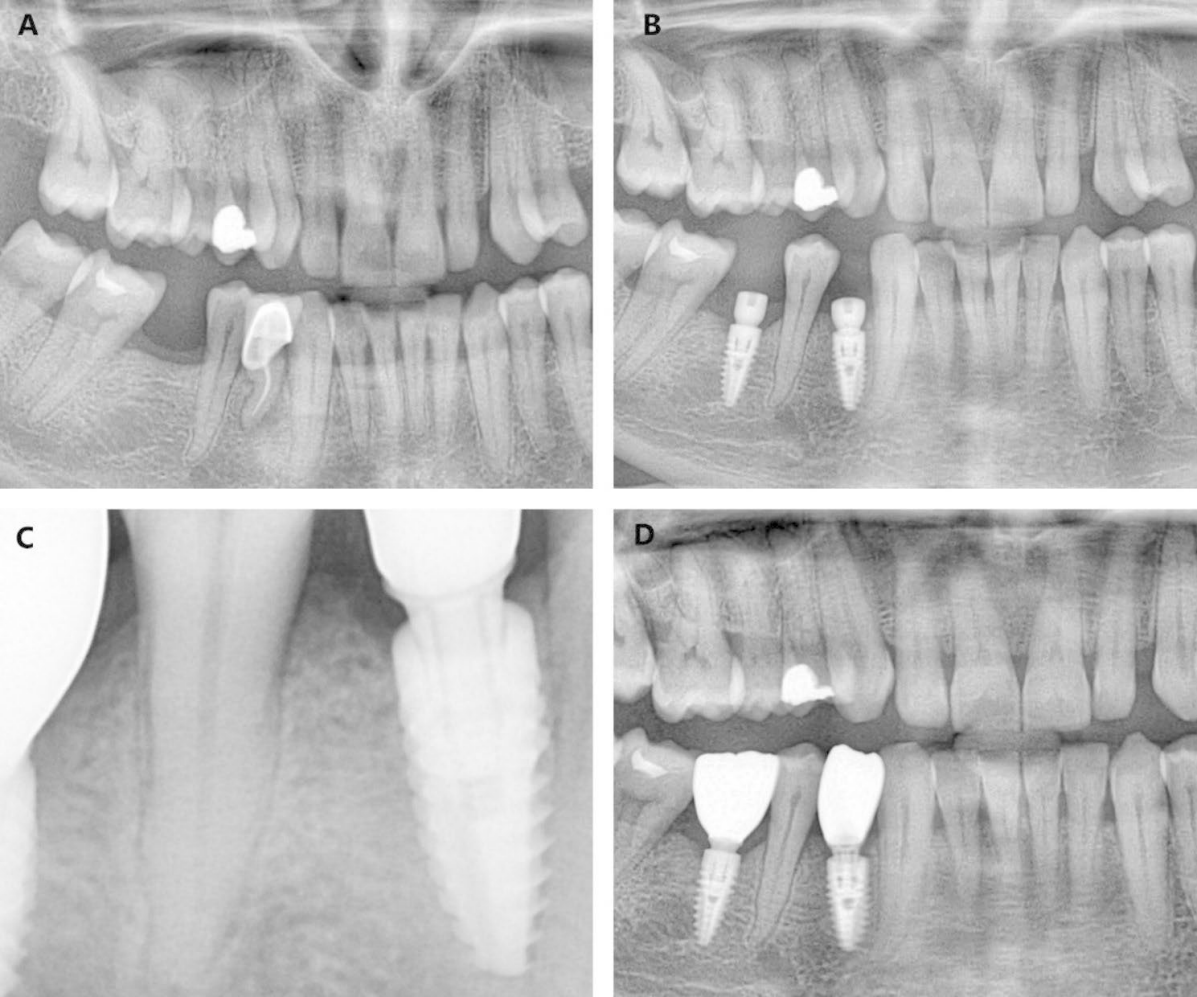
(A) Right mandibular first premolar with periapical pathology. (B) Placement of the implant after tooth extraction and curettage of the socket. (C) Periapical radiography after completion of prosthetic restoration. (D) 18. Monthly control radiograph
